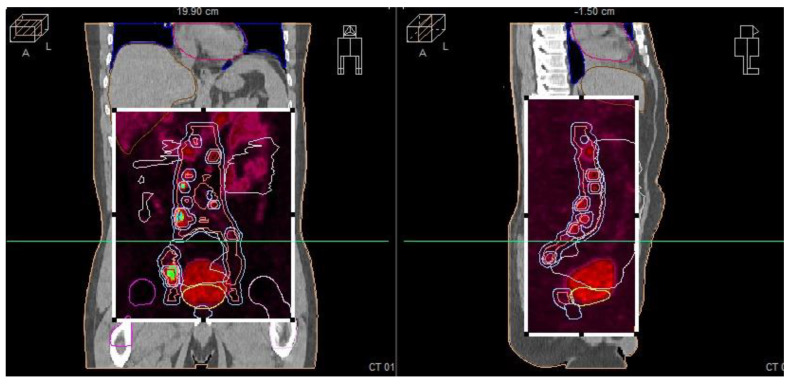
Radiotherapy treatment planning—PET-CT—showing metastatic sites in the prostate and lymph nodes.
